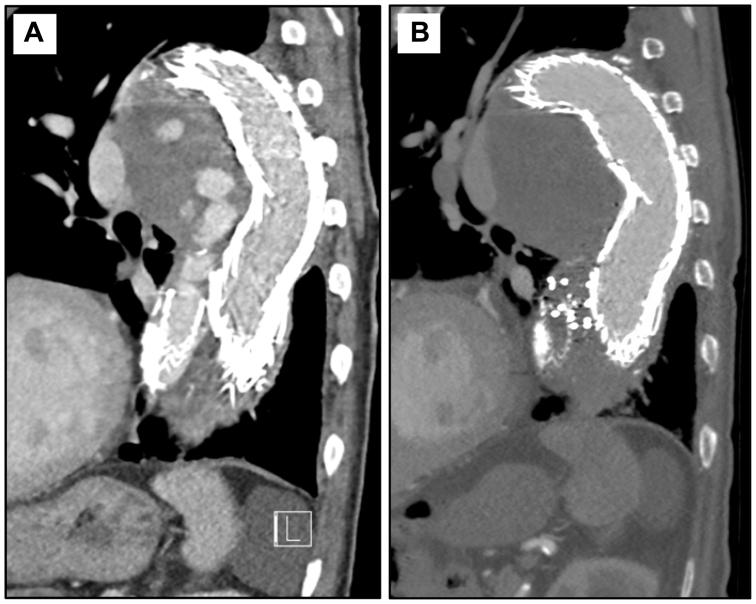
Case 1 computed tomography angiograms. (A) Preoperative sagittal view of the false lumen (FL) with extensive endoleak noted. (B) Six-month postoperative sagittal view at the same level, demonstrating resolution of the endoleak and thrombosis of sac.
